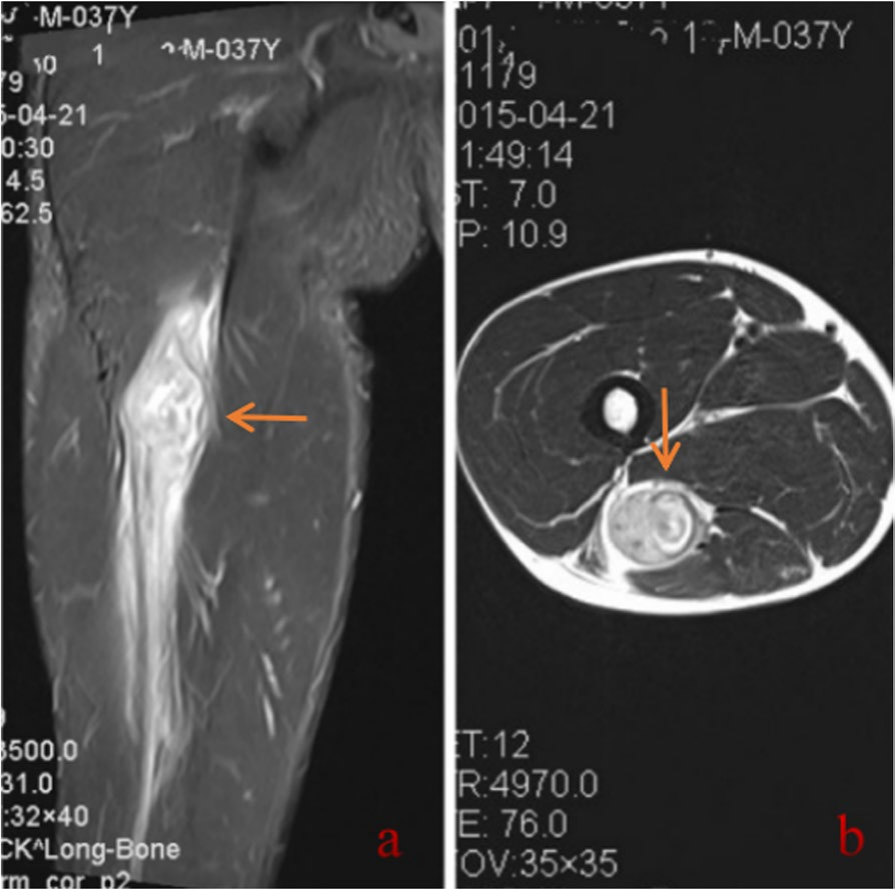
Gluteal MRI in case 2. a Coronal MRI shows a fusiform tumour in the femoral region (arrow). b T2-weighted axial MRI shows a mixed low- and high-intensity signal (arrow)
